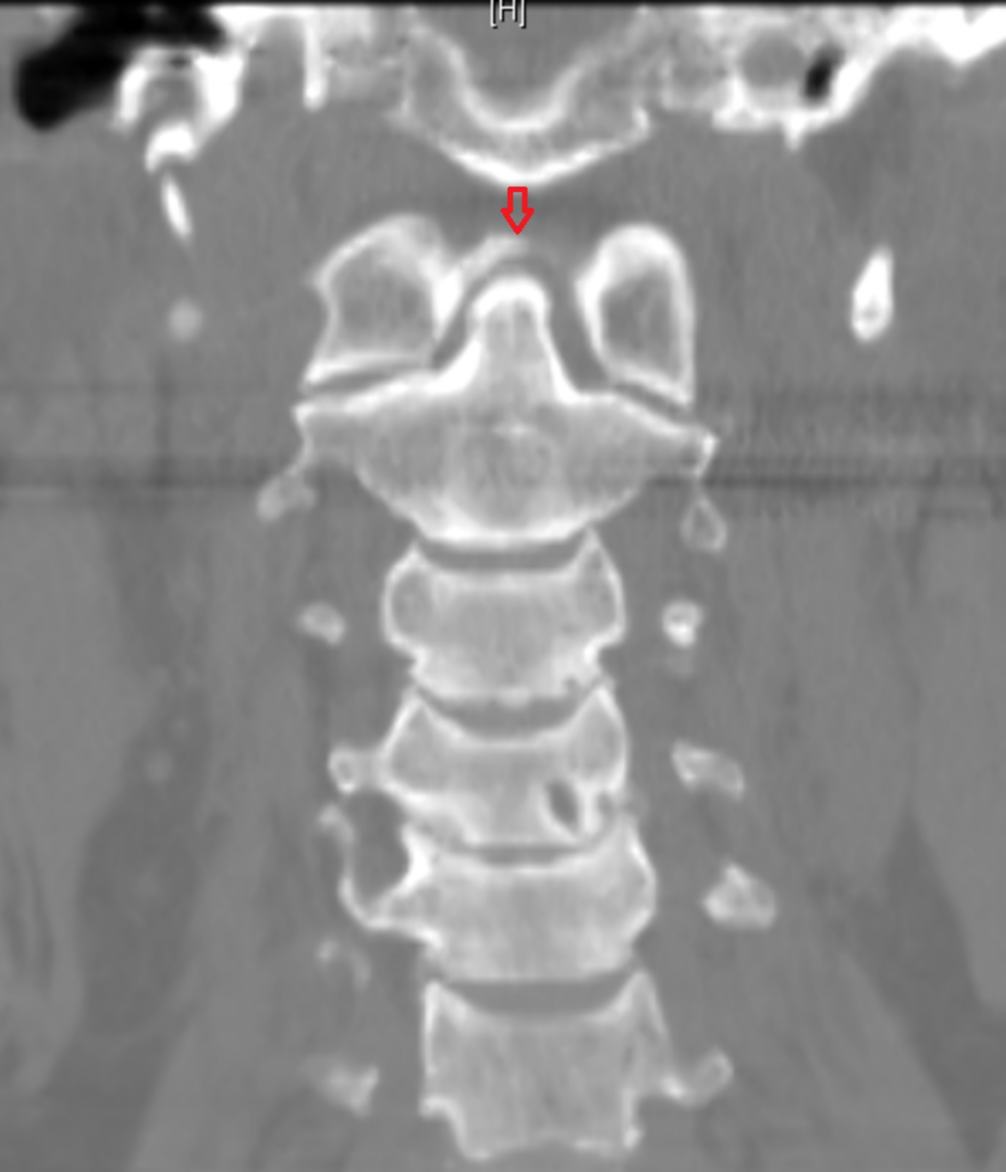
Coronal CT section of the neck showing chondrocalcinosis of the transverse ligament of the atlas The arrow highlights area of calcinosis shaped like a crown or halo around the dens.
